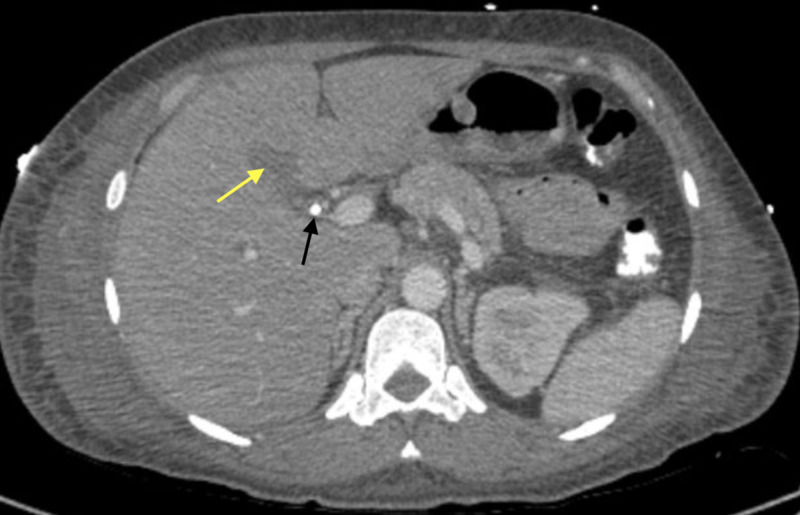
CT Abdomen & Pelvis with Contrast - After Laparoscopic Cholecystectomy & Stent Placement Black arrow: Common bile duct stent Yellow arrow: Decreased fluid collection in the gallbladder fossa
